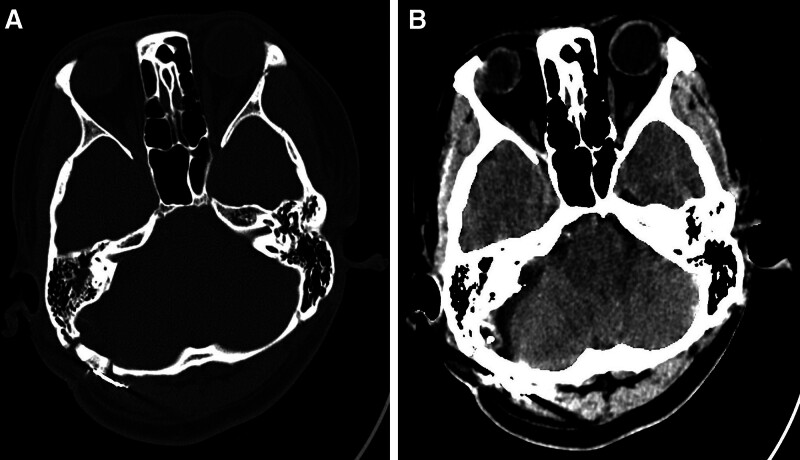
(A and B) Are postoperative computer tomography (CT) scan showing no bleeding or infarction.
